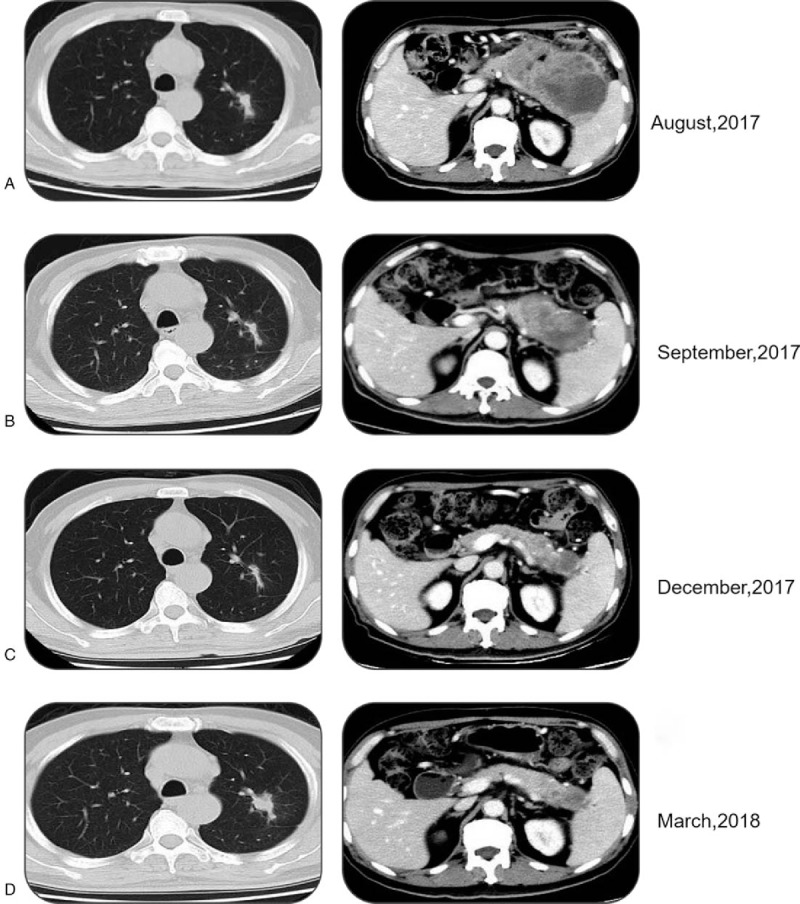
CT scans at baseline of Olaparib treatment (A), after 2 months of Olaparib treatment (B), after 4 months of Olaparib treatment (C), and after 7 months of Olaparib treatment (D).
